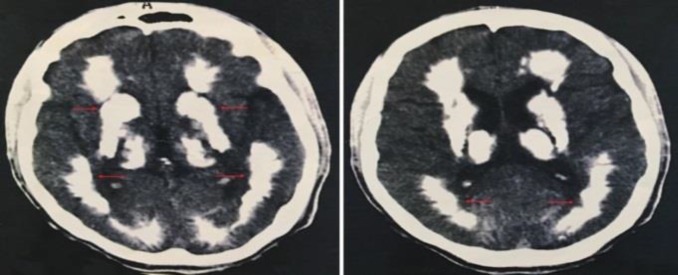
Brain computed tomography scan (CT scan) of the patient with Fahr disease (FD) showing bilateral symmetrical calcifications in the basal ganglia and the cerebral cortex (Arrows)
